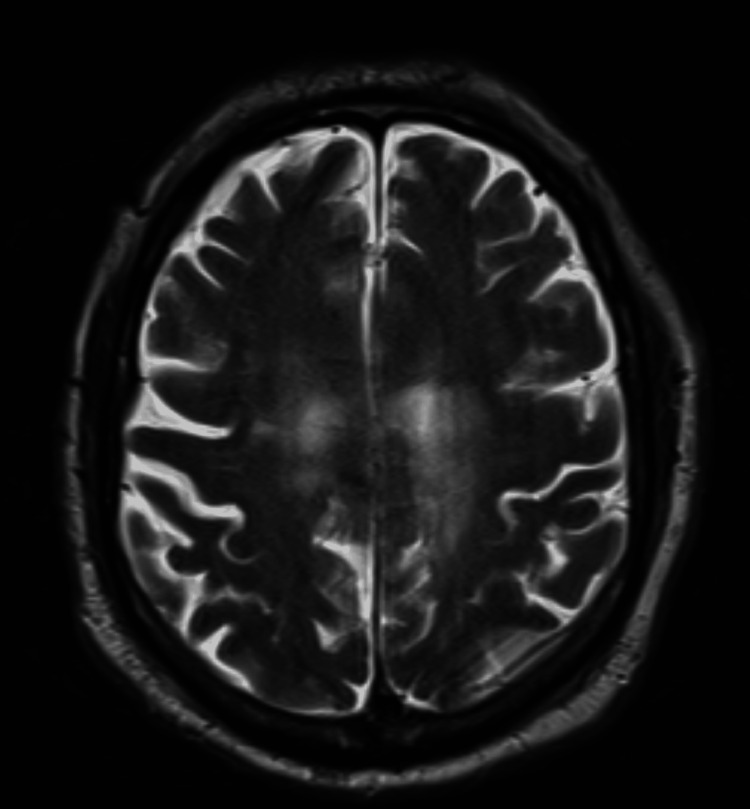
MRI brain without contrast MRI shows no acute infarct, hematoma, mass, extra-axial collection, or hydrocephalus. There is a chronic infarct in the paramedian posterior left superior frontal gyrus, extending into the pre- and postcentral gyri. Mild chronic microvascular ischemic changes are present. Foci of GRE dephasing in the left lentiform nucleus and right cerebellum suggest sequelae of prior hypertensive ischemia. There is generalized parenchymal volume loss. Normal flow-voids are observed in the major intracranial vessels. The sellar and pineal regions are unremarkable, and the craniovertebral junction is intact. GRE: gradient-recalled echo
